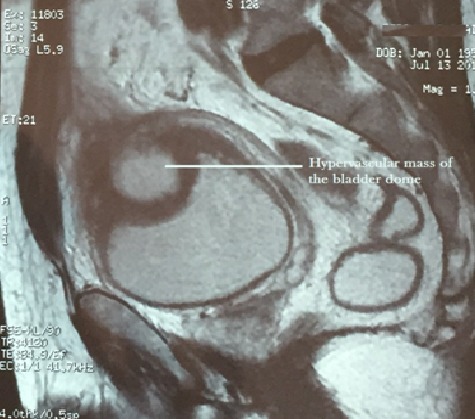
Sagittal section in MRI T2 showing the CHB
